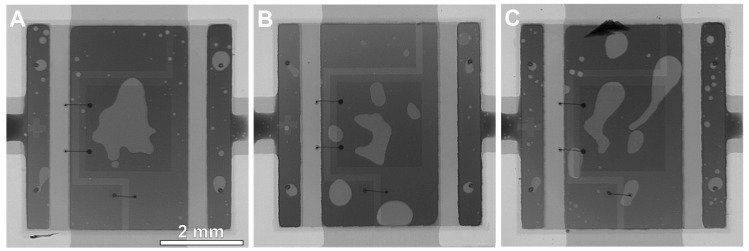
X-ray images of the solder joints under the LED component: (A) reference SACX0307 sample; (B) SACX0307-nanoTiO_2_ sample; (C) SACX0307-TiO_2_ sample.
